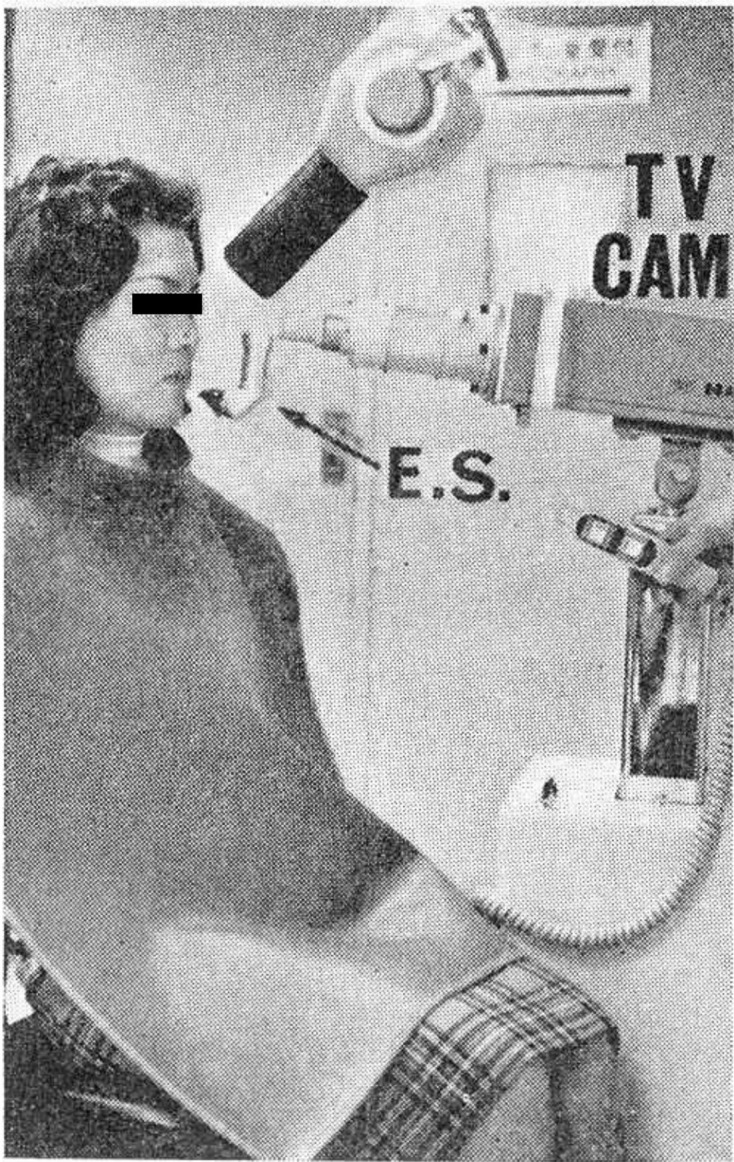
Intraoral X-ray TV system. *Note*. Reprinted from “Real Time radiological survey by intraoral fluoroscopic TV system to minimize radiation dose”, by Ando S, et al., *Dental Radiology* 1978; 18:125, Figure 2.
